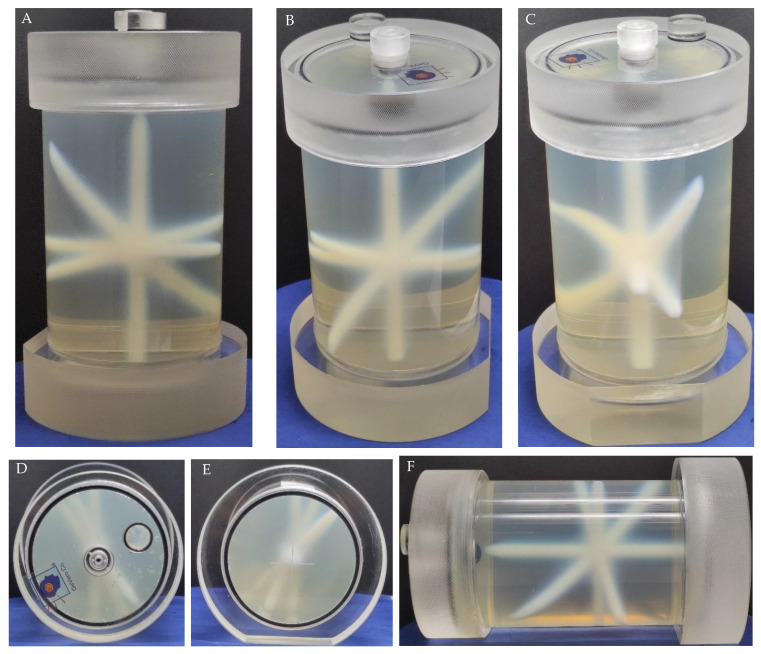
Isocenter determination: 3D option. PABIG^nx^ in PH-5-DD1 GeVero Co. container was irradiated (A–F) and scanned with a TrueBeam accelerator (iCBCT, mode: Pelvis, mean over 3 series). The photographs in (A–C) are for the rotated sample ((A): 0°, (B): 180° and (C): 270°) to better inspect of the irradiation pattern; (D,E) are the top and bottom views, respectively, and (F) is the side view.
